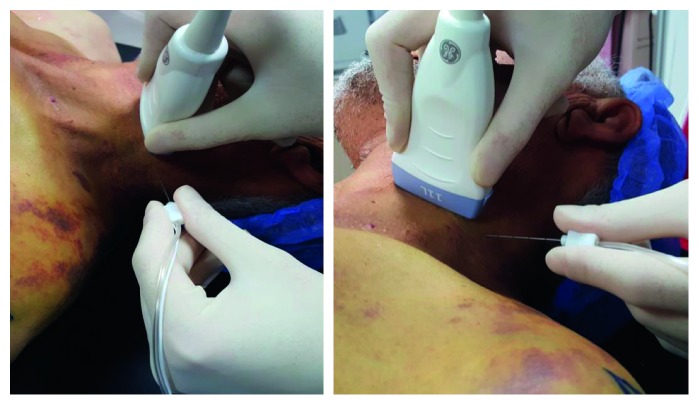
Position of the ultrasound transducer and the needle during the performance of blocks.
